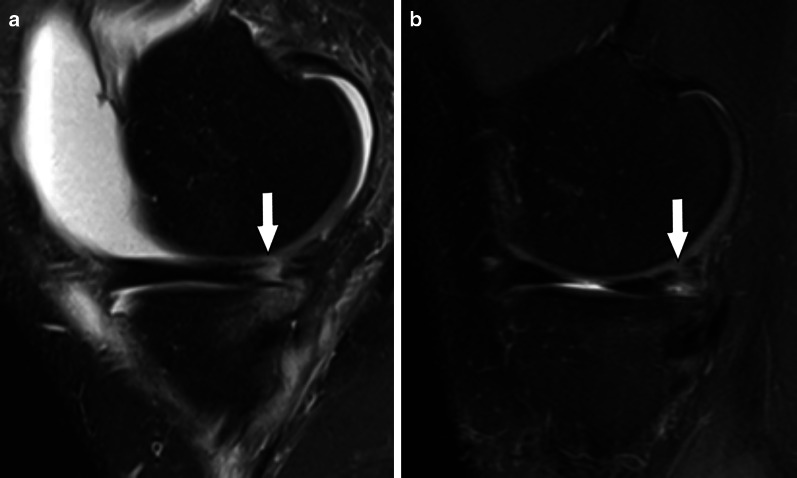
(a) Sagittal fat-suppressed MR image shows a type 4 acute ramp lesion (arrow) and in (b) the postoperative appearance after 4 months of surgery (arrow)
